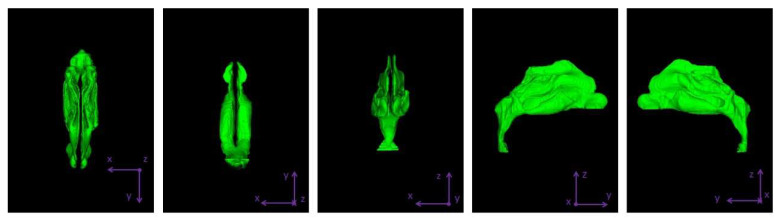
The 3D geometry of the nasal and nasopharyngeal cavities shown in various planes. The software used to segment the geometry from CT DICOM data was ITK-SNAP [20].
